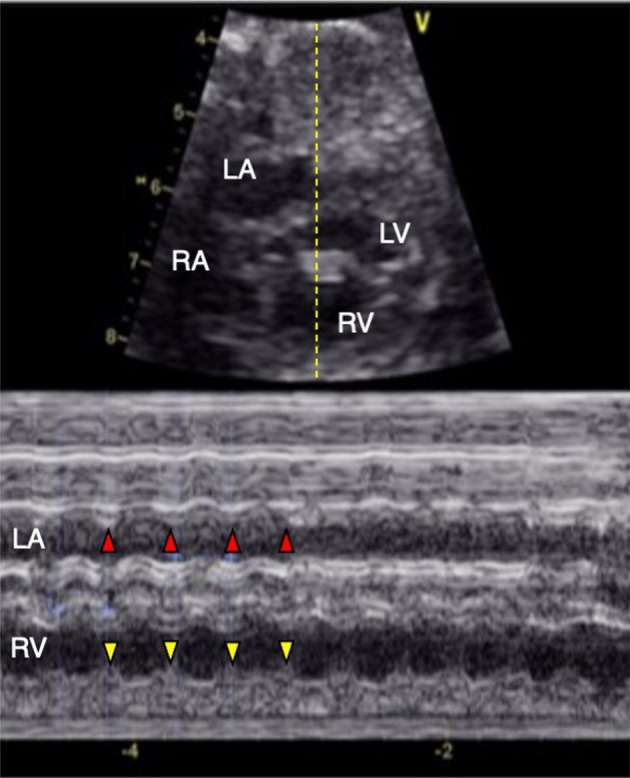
Fetal echocardiogram. 2D mode (upper) shows the ultrasound line used for M-mode (lower) echocardiogram crossing the left atrium (LA) and the right ventricle (RV). M-mode shows tachycardia with a heart rate at 190 beats per minute. LA left atrium, LV left ventricle, RA right atrium, RV right ventricle.
